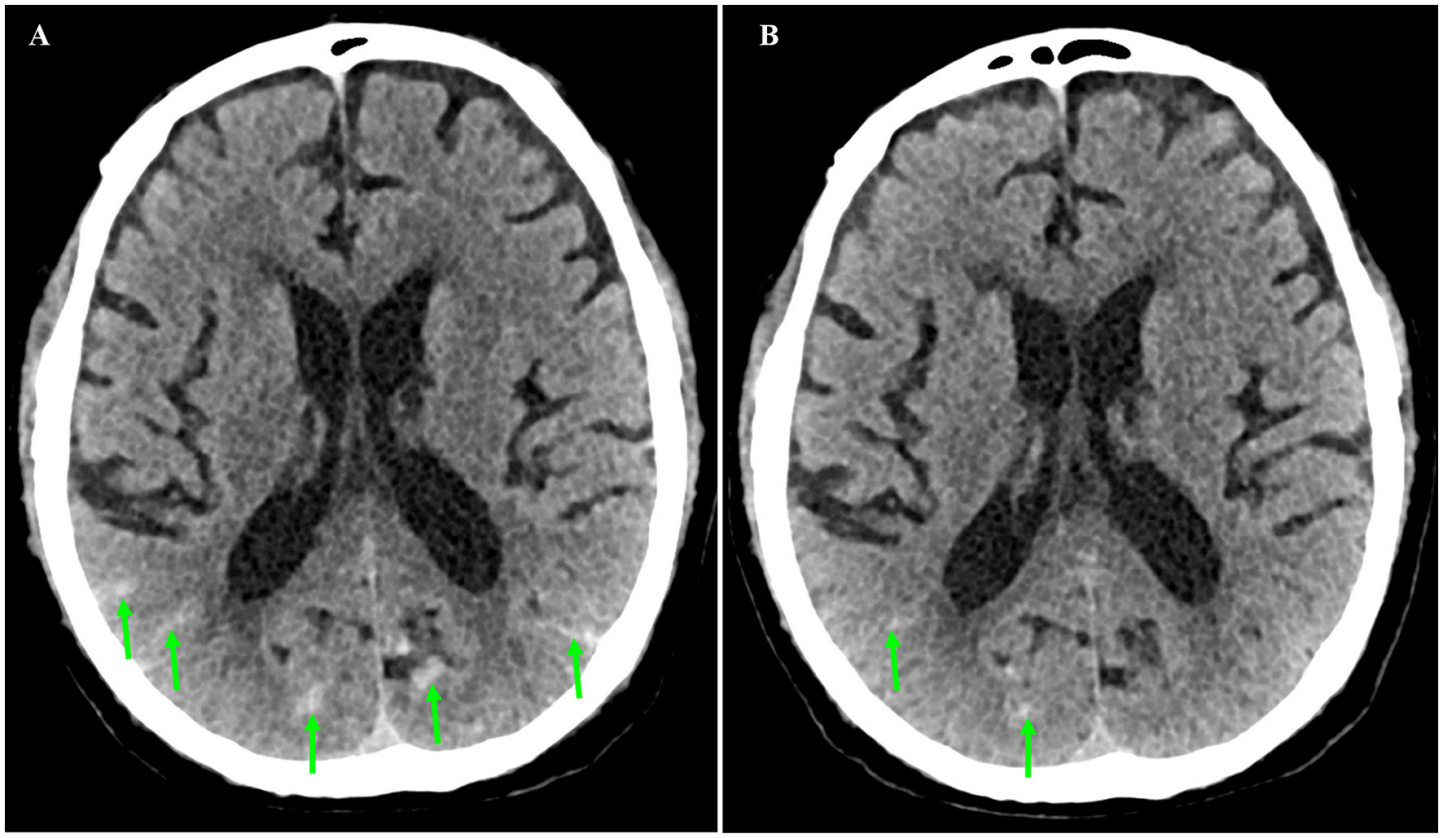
Axial sections of non-contrast brain computed tomography. (A) Subarachnoid hemorrhage was present in the right and left parietal and occipital lobes (arrow) on hospital day 12 (February 24, 2020). (B) Subarachnoid hemorrhage was almost absorbed on hospital day 23 (March 6, 2020).
